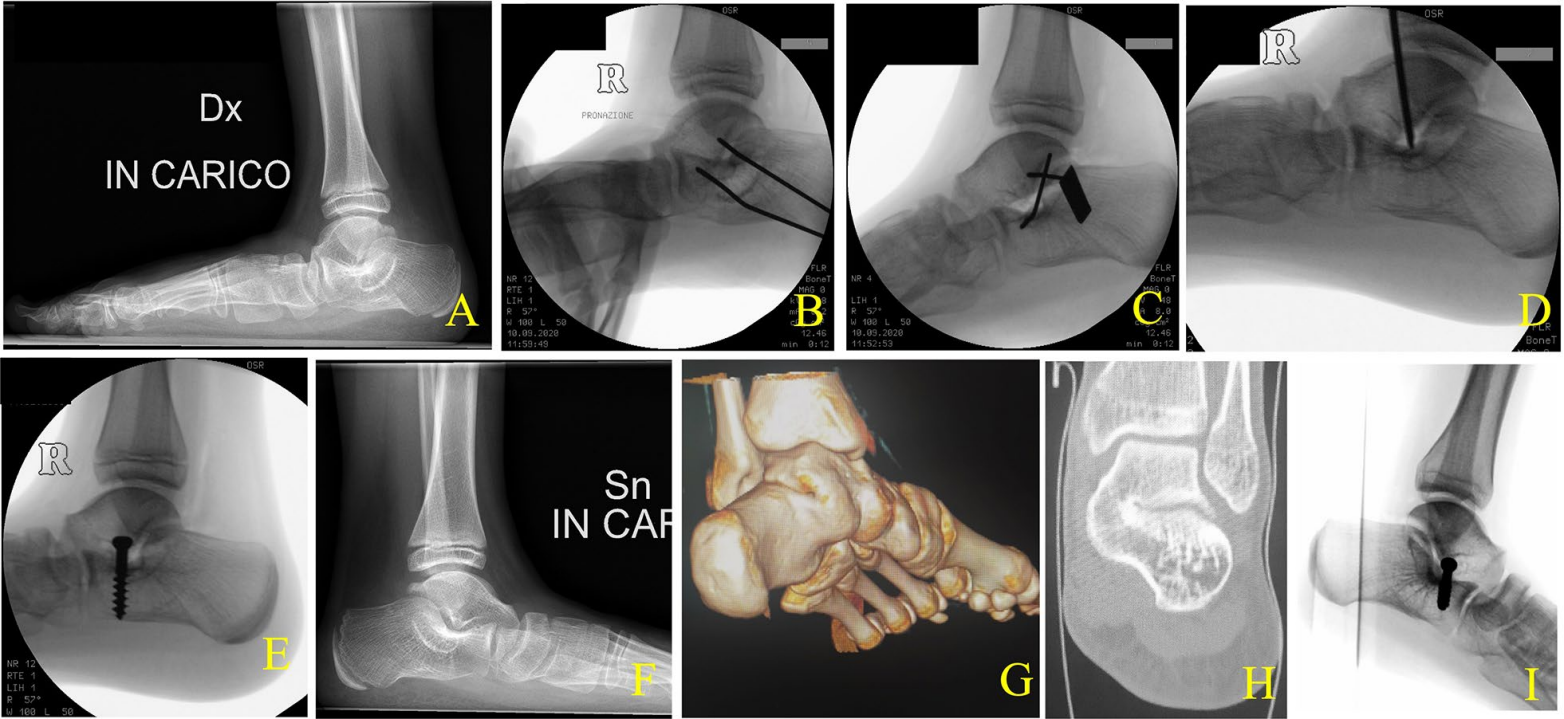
Adolescent affected by bilateral TCC A Preoperative weight bearing lateral view x-ray of the right foot with a rigid symptomatic flatfoot deformity in a 12-year-old boy. B x-Ray during coalition resection showing the two parallel distractor pins into the talus and calcaneus, respectively. C x-Ray after resection showing the achieved mobility of the subtalar joint. See the divergent distractor pins. D SESA procedure with the *K*-wire showing the correct point for screw insertion into the calcaneus. E Postoperative x-ray after SESA. The correct anatomical talo-calcaneal relationship is maintained. F Weight bearing lateral view x-ray of the left foot with a rigid asymptomatic flatfoot deformity of the same patient at 12 years of age. G 3D-CT reconstruction at 16 years of age showing a complete osseous coalition of the rigid left foot, which has become symptomatic. H CT slice showing the dimension of the coalition. I Postoperative x-ray after coalition resection and SESA
